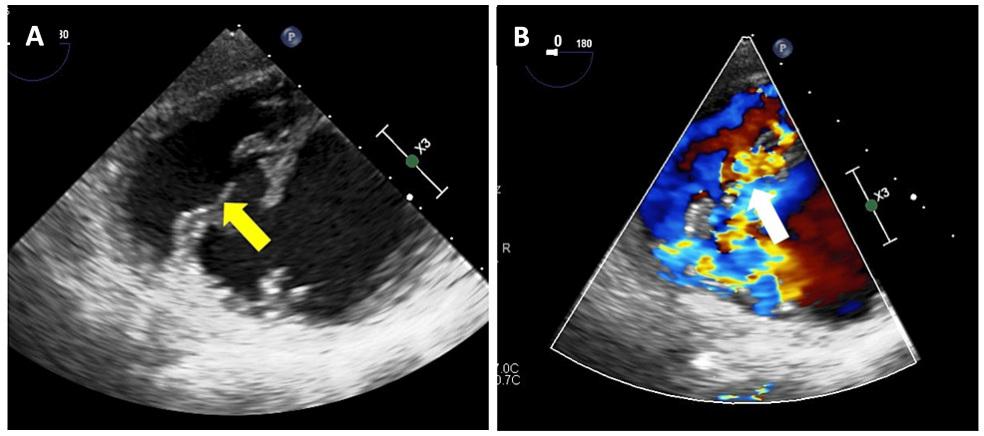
Transesophageal echocardiography. (A) Large and complex ventricular septal rupture (VSR) of 17 mm (yellow arrow). (B) Color Doppler in the area of the VSR with a left-to-right shunt (white arrow).
